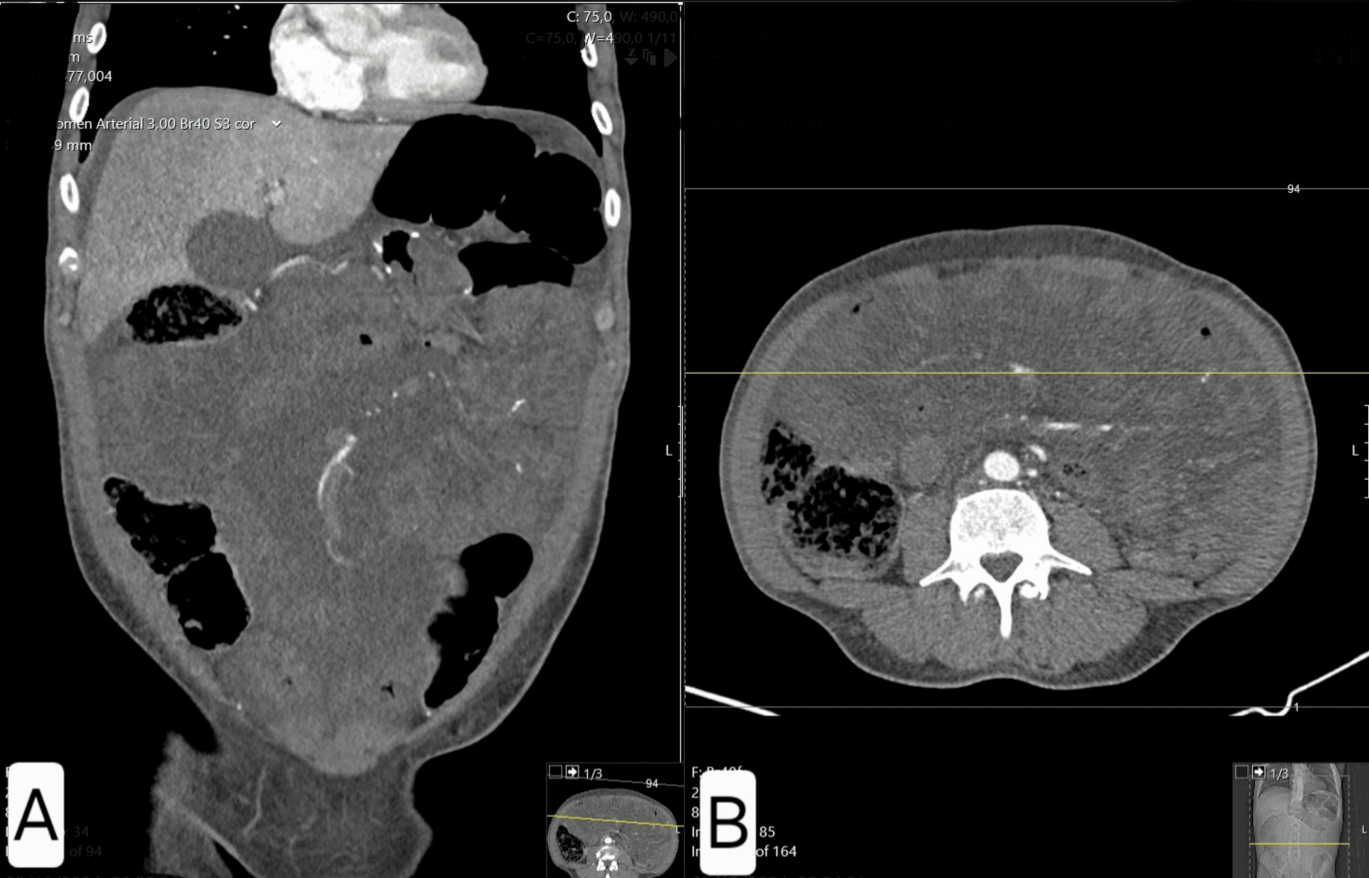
Case 6 - CT findings suggesting extensive small bowel hypoperfusion Image A (coronal view): Distended small bowel loops with global hypoenhancement and mural thickening. Image B (axial view): Reduced contrast enhancement throughout the mid-abdominal small bowel, raising suspicion of diffuse ischemia. These findings were corroborated intraoperatively and prompted urgent surgical intervention.
